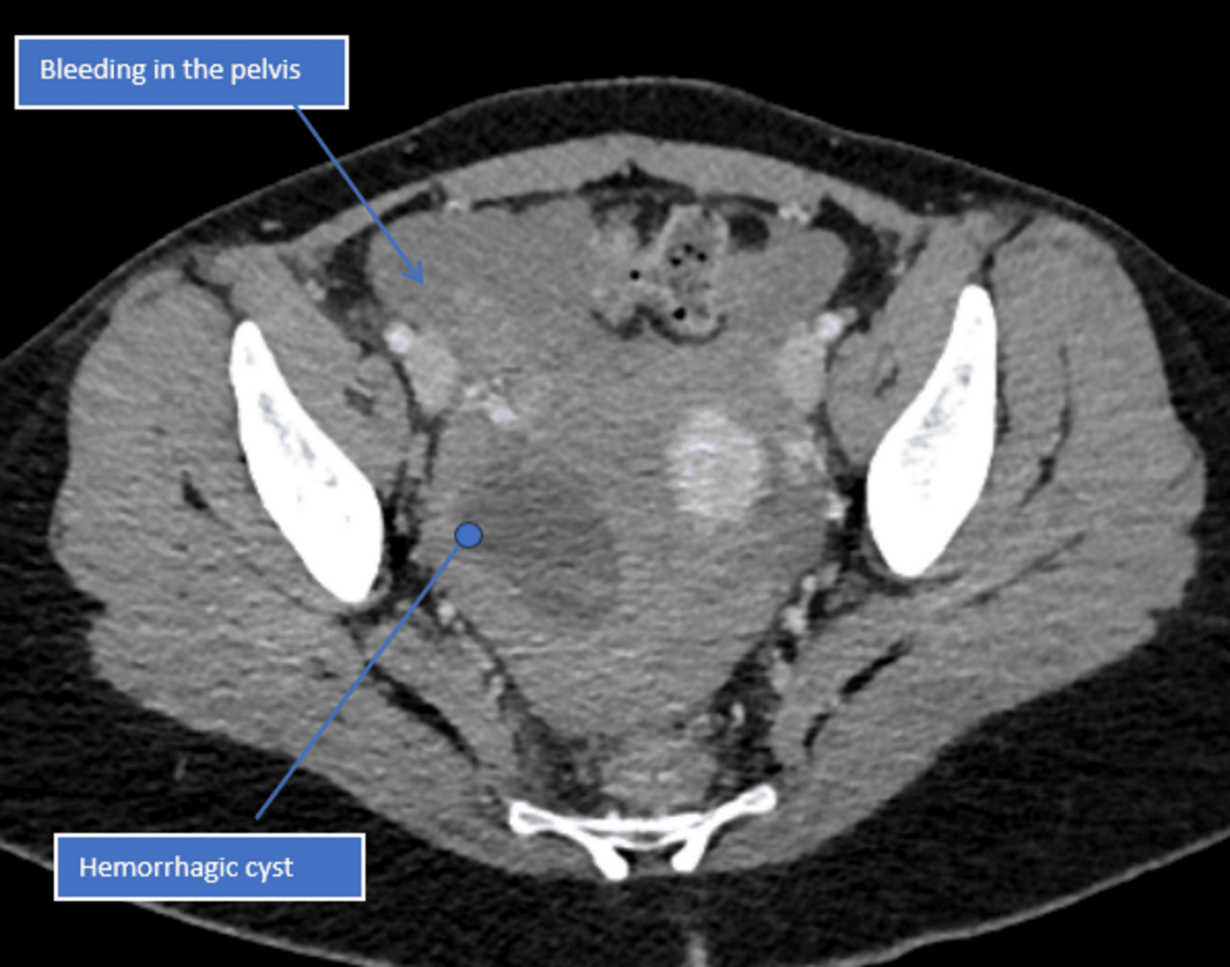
CT pelvis shows the cyst and the intra-abdominal bleed (arrows)
